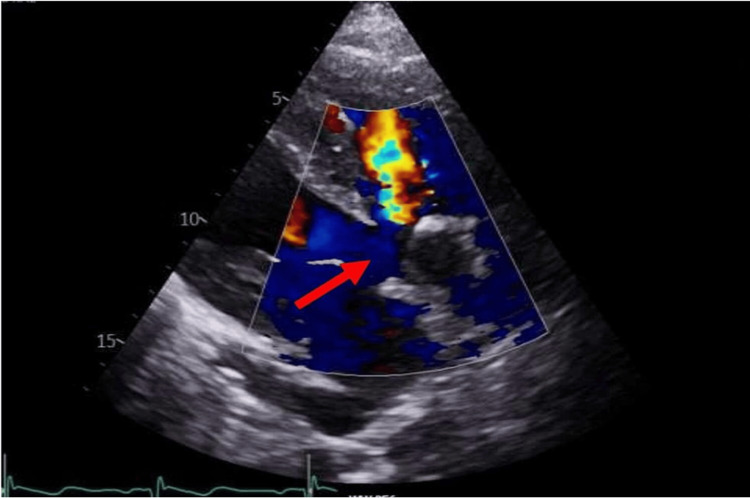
ECHO image in parasternal long axis view (PLAX) across the septal defect. Red arrow shows membranous ventricular (RV) septal defect with overriding aorta and moderate jet of aortic regurgitation directed to the right ventricular cavity Furthermore, moderate aortic regurgitation was noted. There was no indication of significant RV dilatation or hypertrophy. Despite established pulmonary hypertension, we couldn’t accurately estimate pulmonary artery systolic pressure (PASP) due to pulmonary atresia
